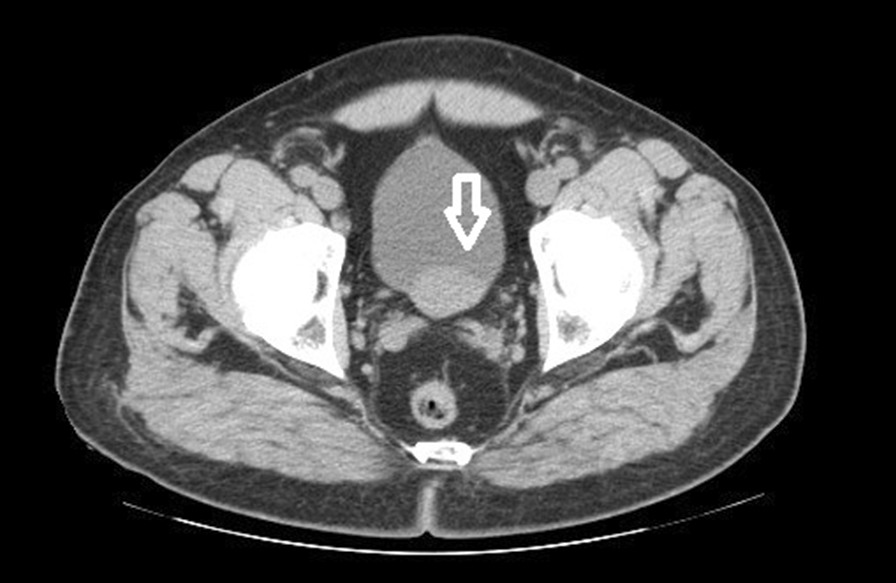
CT confirmed the presence of a solid, well-delineated mass measuring 41 × 24 mm that appeared to arise from the bladder trigone wall
